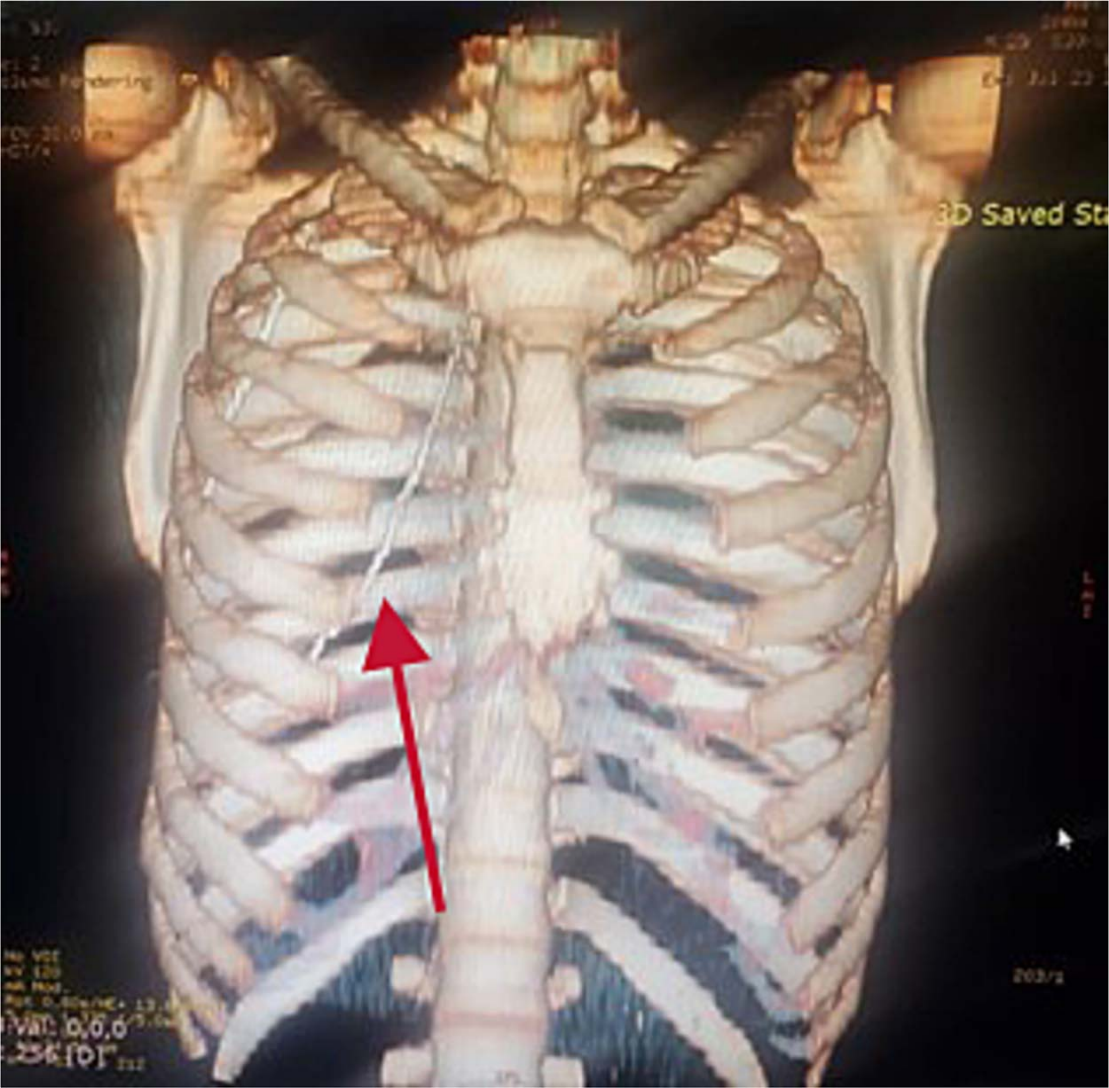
Reconstructed 3D image of the CT scan.
